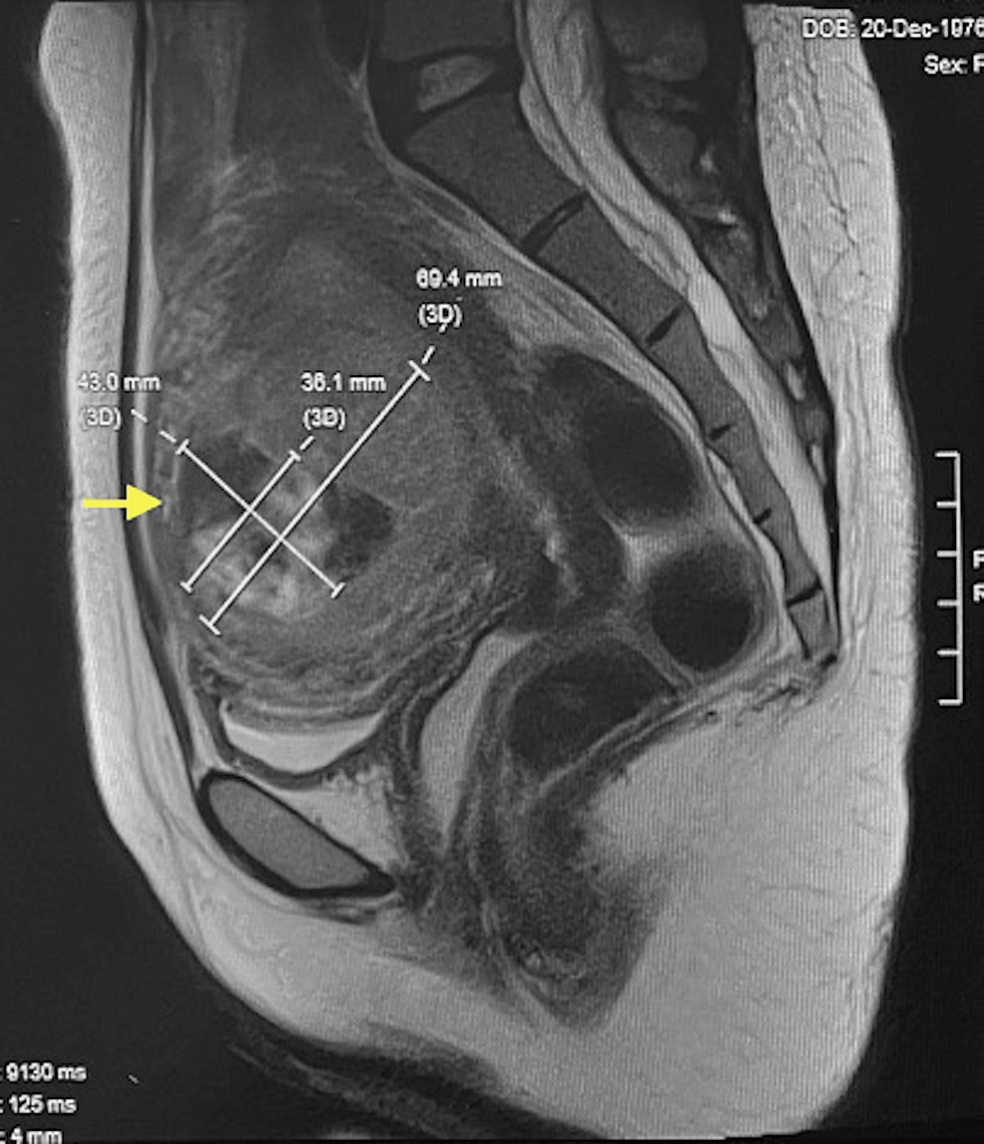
MRI four-month after HIFU HIFU: High-intensity focused ultrasound MRI four-month after HIFU showed a minimally reduced fibroid size (arrow).
